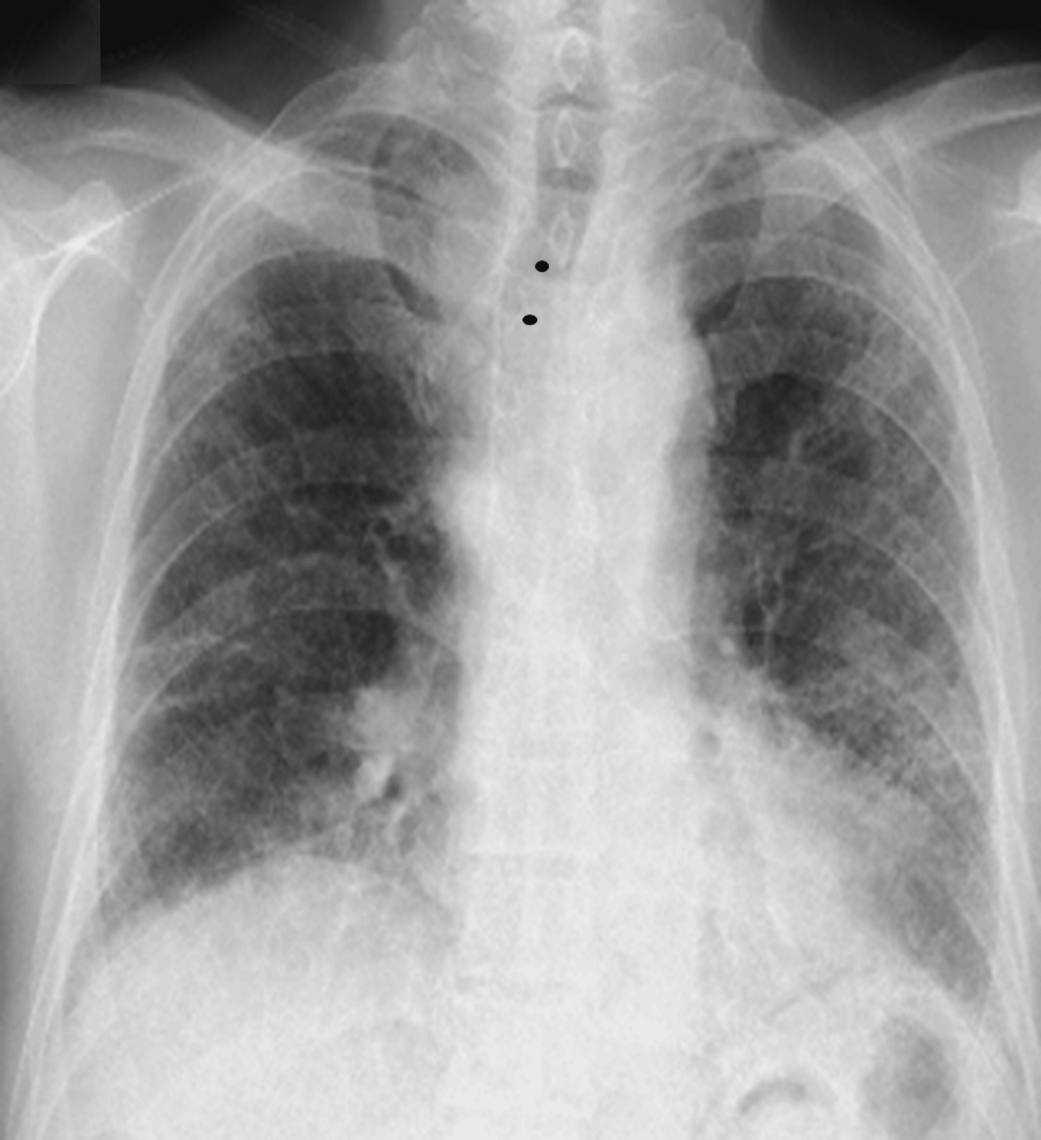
Chest X-ray at initial visit A chest X-ray showed volume loss, tracheal deviation (black circles), and bilateral reticulation predominantly in the lower lung.
